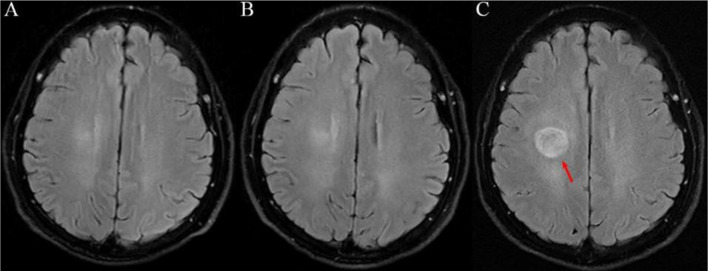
Serial brain MRI findings. (A) Preoperative MRI (November 14, 2022) showing no metastasis. (B) Follow-up MRI (April 12, 2024), negative. (C) MRI (November 6, 2024). T2 FLAIR image showing a new solitary lesion in the right centrum semiovale, 2.5 cm × 2.0 cm (arrow).
